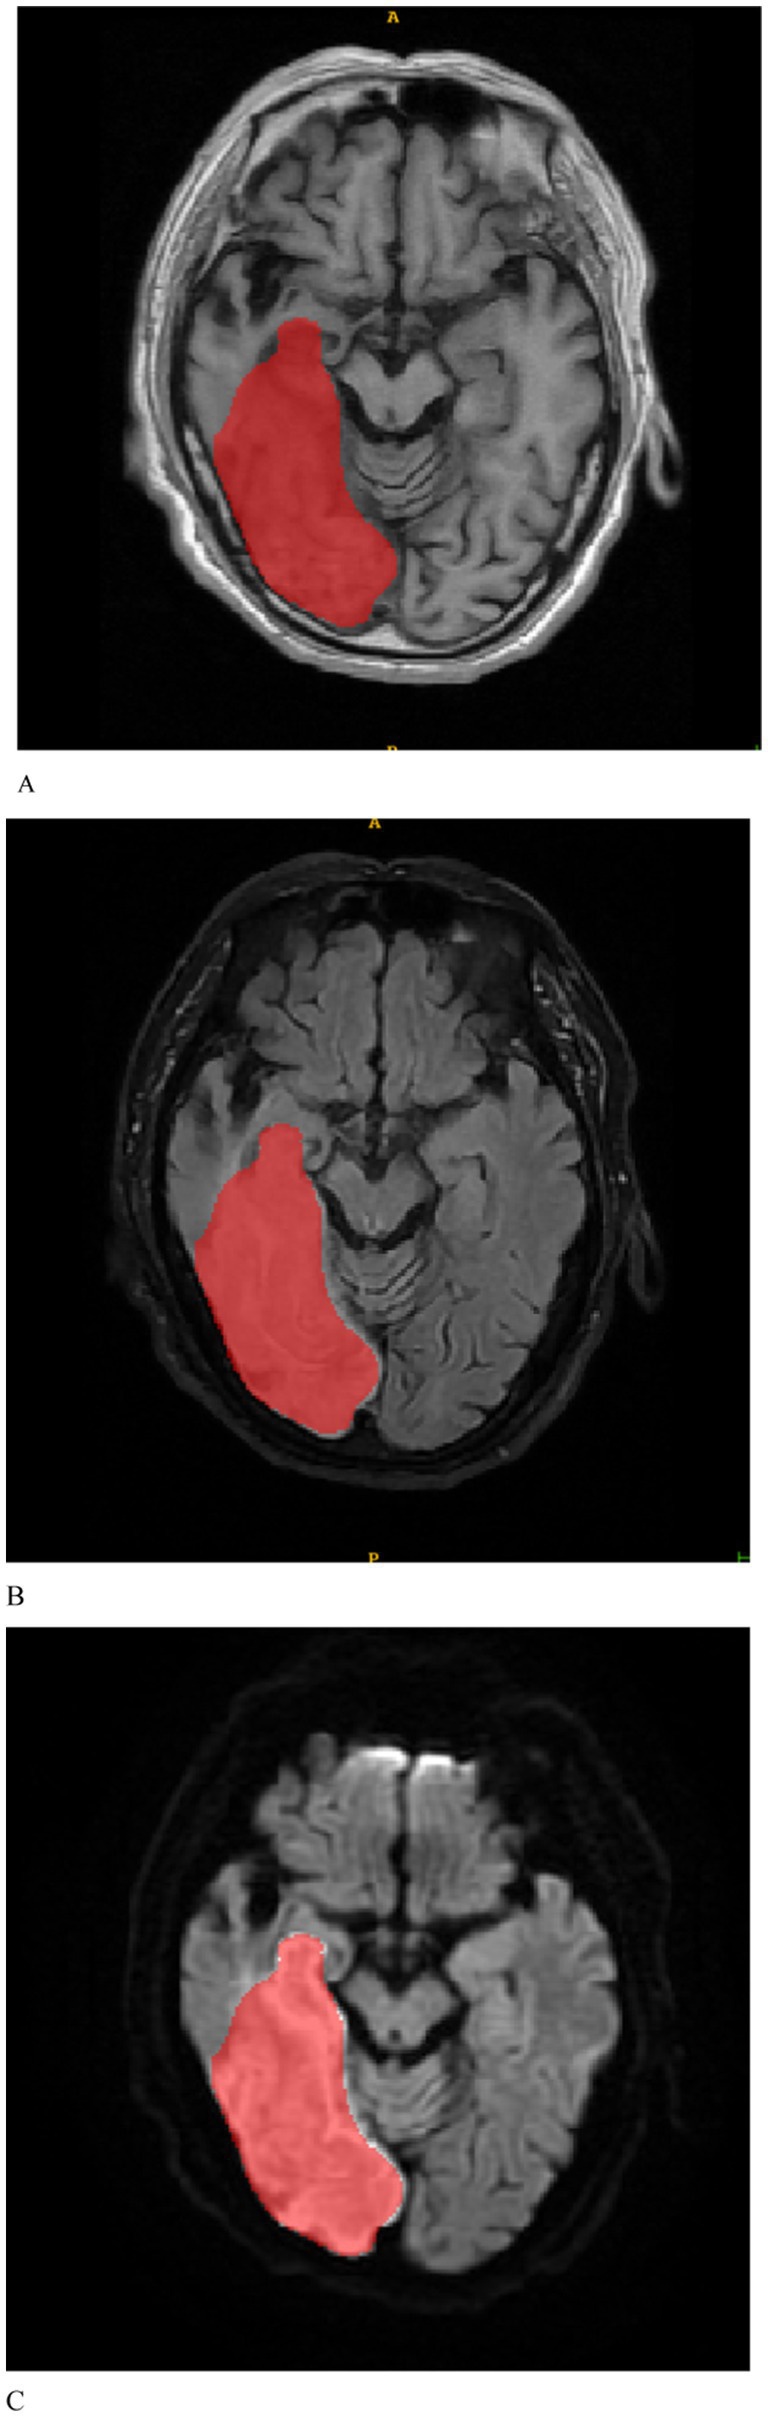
Based on the manually delineated regions of interest for patients with stroke, (A–C) represent the T1WI, FLAIR, and DWI sequences, respectively.
